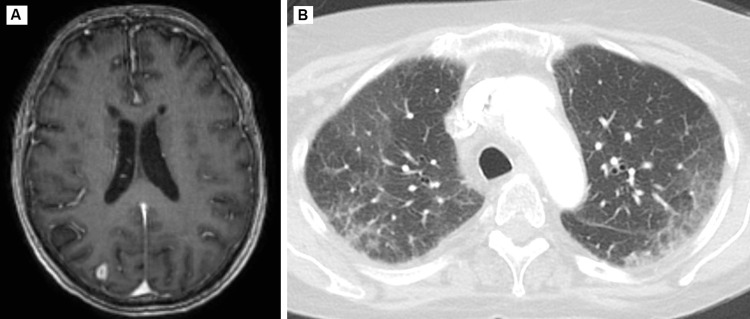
MRI of the brain (A) and CT scan of the chest (B) one month after discharge showing an overall decrease in lesion size and ground-glass opacities. MRI: magnetic resonance imaging; CT: computed tomography
